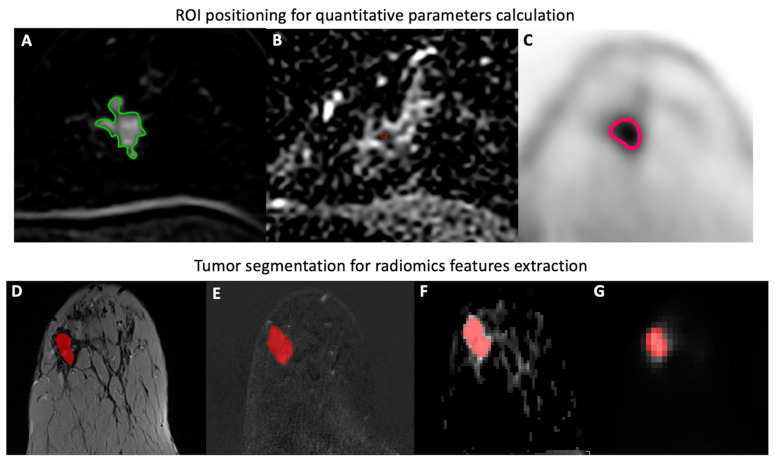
Examples of 2D ROI placement for the extraction of quantitative parameters (mean transit time; plasma flow; volume distribution; ADC mean; and SUVmax, mean, and minimum) (A–C), and whole tumor segmentation for radiomics features (first, second, and higher order) extraction (D–G) from primary BC tumor lesions on DCE (A,E), DWI (B,F), PET (C,G), and T2-weighted (D) images.
